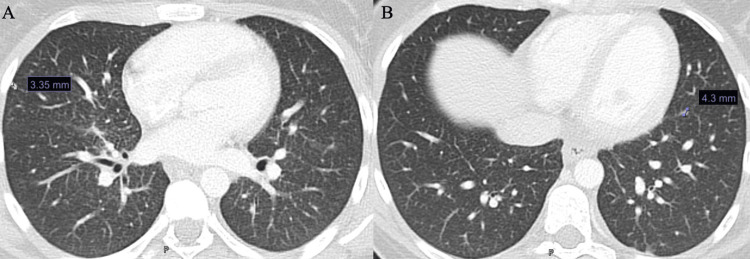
Computed Tomography (CT) of the Chest CT of the chest showing small pulmonary nodules. One 3.35 mm nodule is present in the right middle lobe, and one 4.3 mm nodule is seen along the left lung fissure (labeled in A and B).
